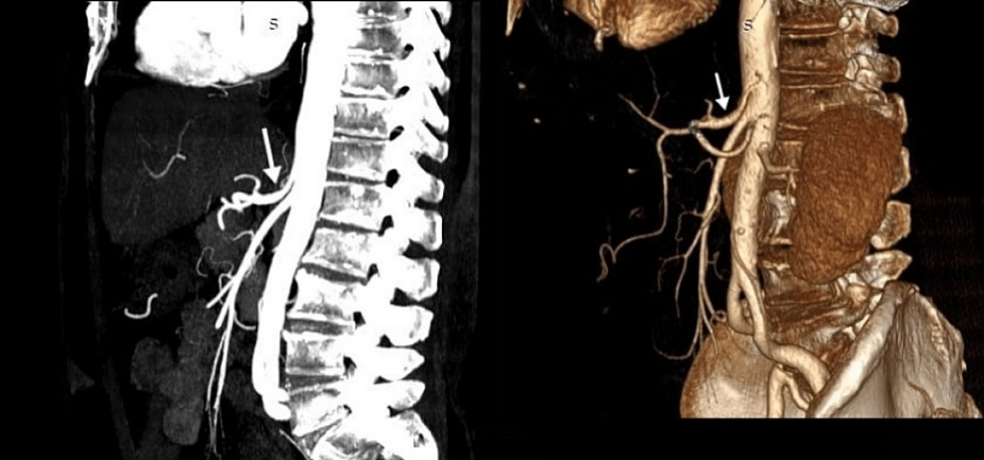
Computed tomography angiography of the abdomen and pelvis (sagittal view) with two white arrows showing the compression of the celiac artery
